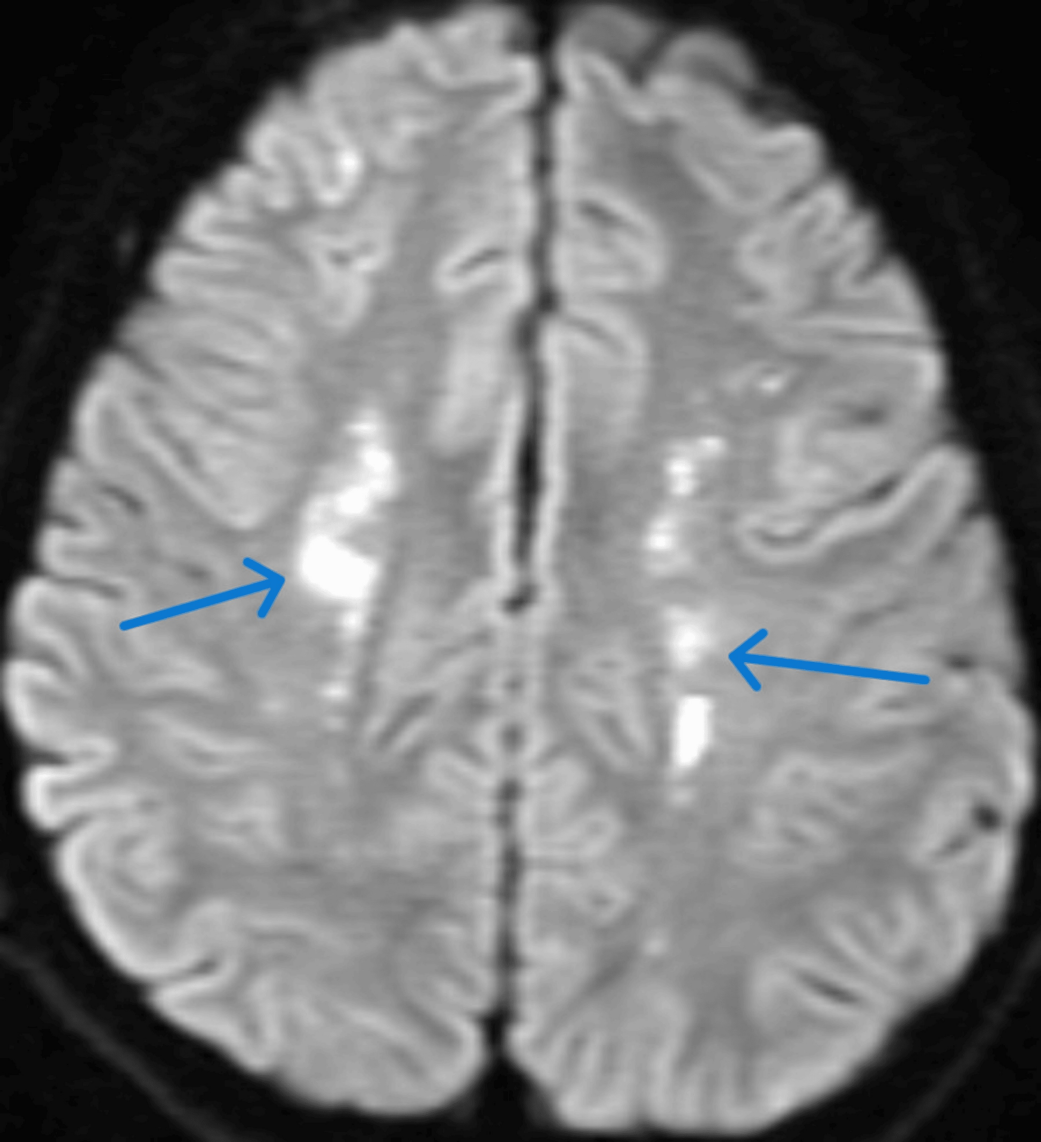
Contrast-enhanced MRI brain DWI sequence showing diffusion restriction at parieto-temporal areas suggestive of small vessel vasculitis
